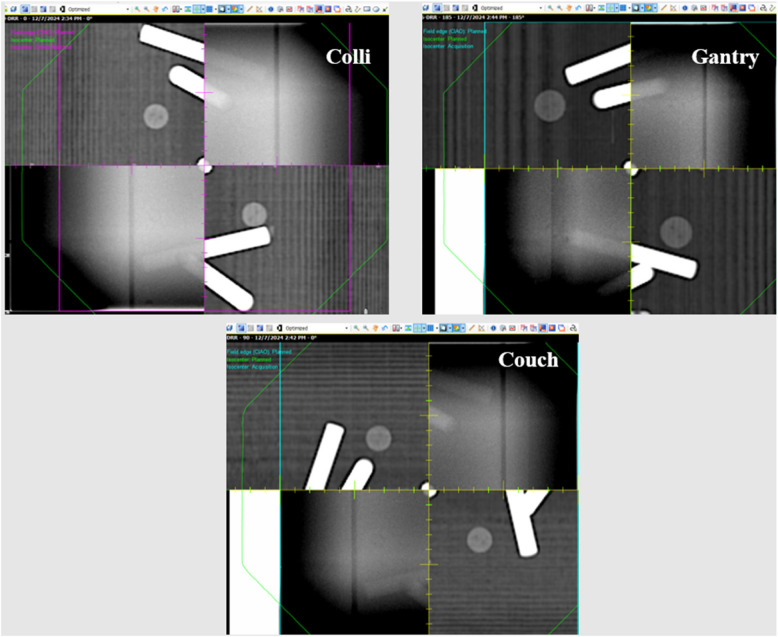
Analysis of Winston-Lutz test (matching of MV image with DRR generated from the planning CT)
